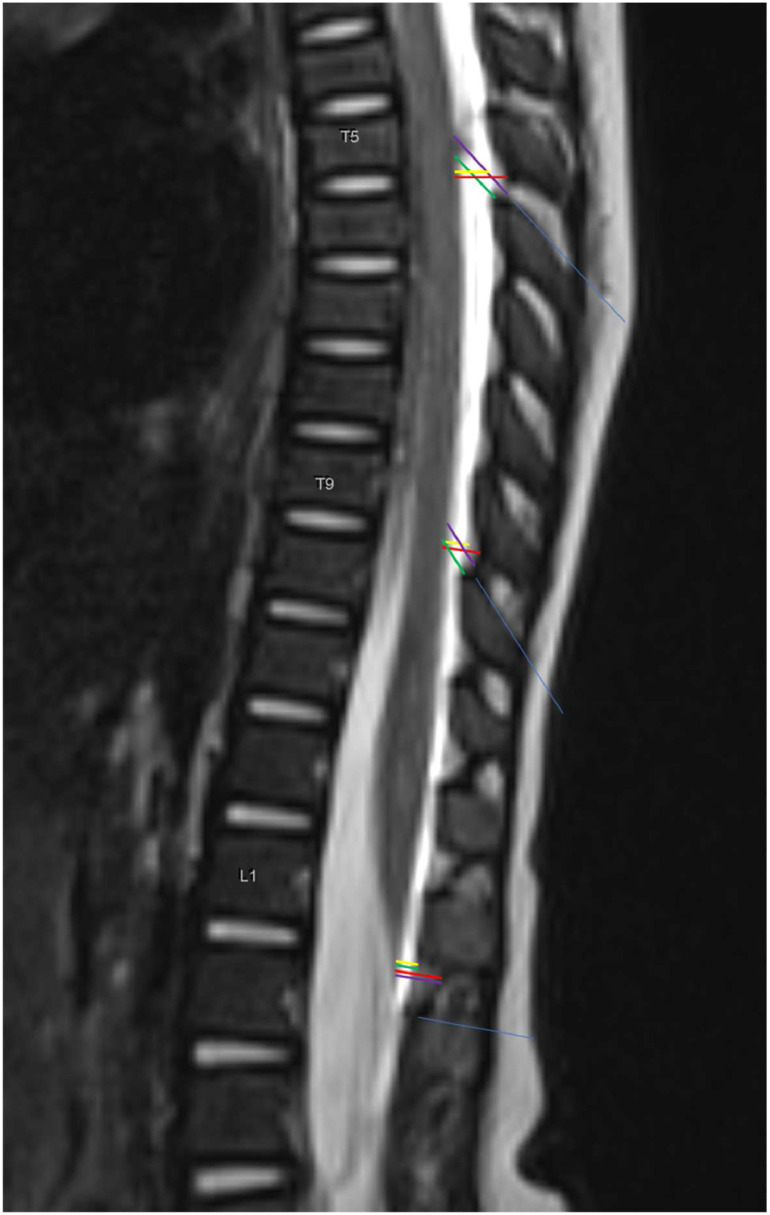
T2-weighted sagittal MRI spine image demonstrating 4 measurements/distances measured at levels T5/T6, T9/T10, and L1/L2: (1) dura-SC perpendicular to the SC (yellow line); (2) LF-SC perpendicular to the SC (red line); (3) dura-SC parallel to spinous process (green line); and (4) LF-dura parallel to spinous process (purple line). The long/blue line represents the line parallel to the inferior spinous process used as reference for measurements (3) and (4). Dura, Dura mater; SC, Spinal Cord; LF, Ligamentum Flavum.
